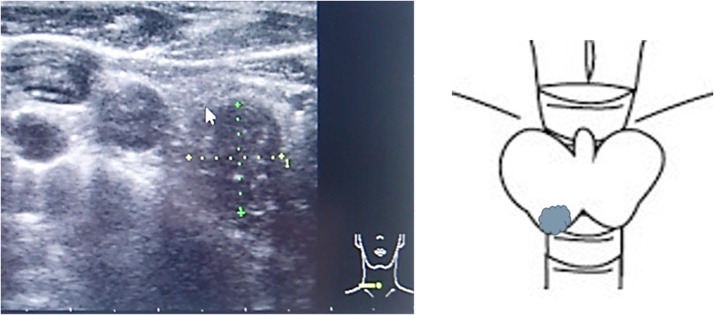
A 12 mm mass is seen in the right lower thyroid lobe with ultrasonography.
